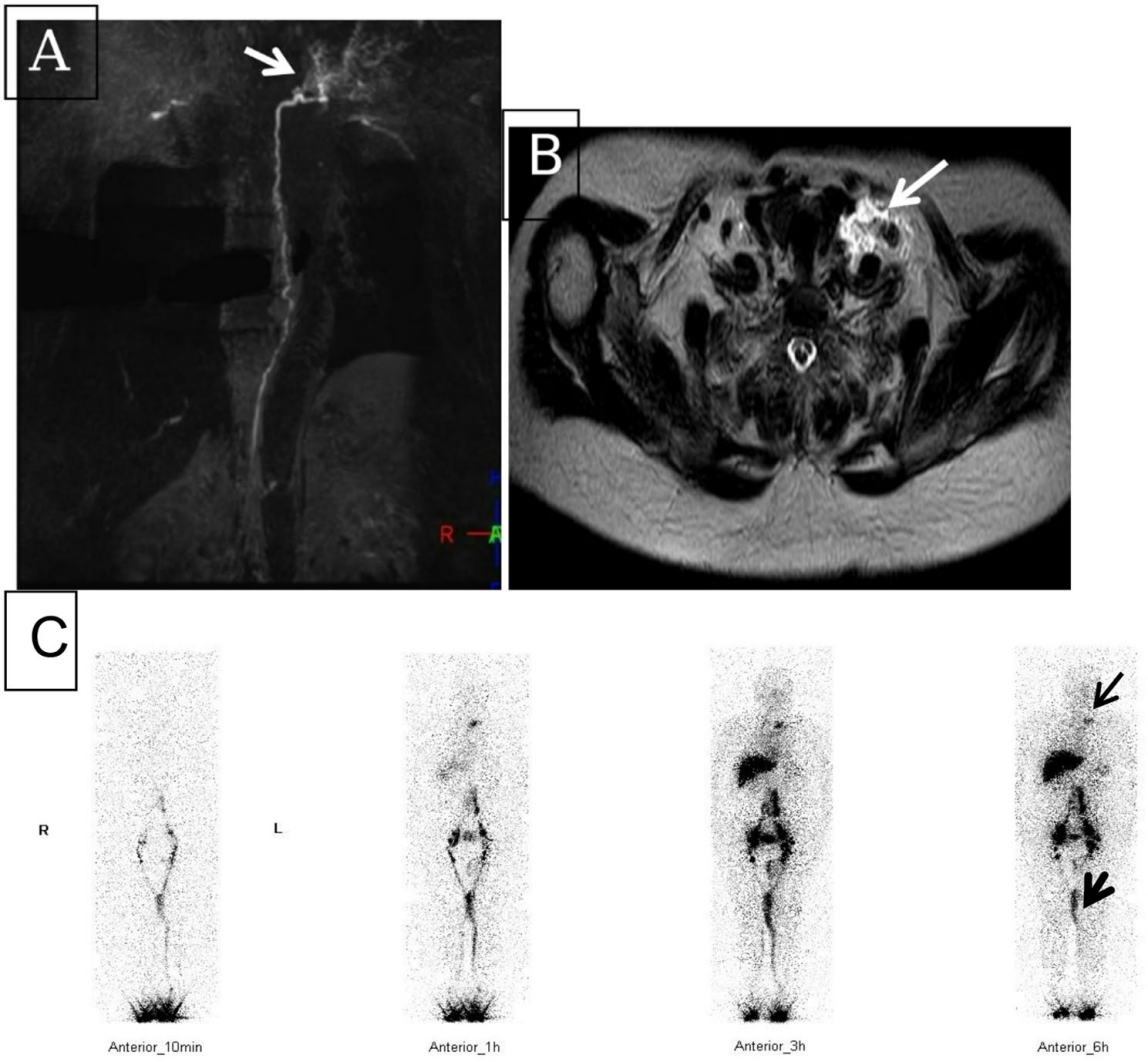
A–C A patient with RA with lymphatic system involvement. (A) Coronal Non-contrast Magnetic Resonance Lymphangiography (NCMRL) showing multiple abnormal small lymphatic vessels (white arrow) in the left jugular venous angle; (B) Axial NCMRL showing multiple abnormal small lymphatic vessels (white arrow) in the left jugular venous angle. (C) ^99^TC^m^-DX lymphoscintigraphy (anterior): continuous visualization of the left venous angle at 1 h, 3 h, and 6 h (thin black arrow); a small amount of contrast agent is retained subcutaneously on the inner side of the left thigh (thick black arrow), indicating lymphedema
